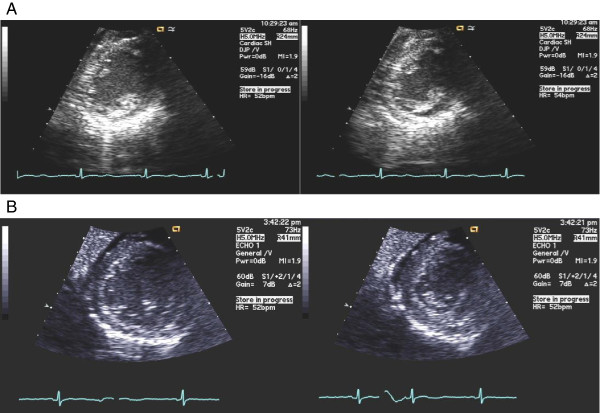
TransThoracic Echocardiogram (TTE). A. Initial TTE: Left ventricular hypokinesis and EF 10-15%. B. Post recovery TTE: EF 40%.
